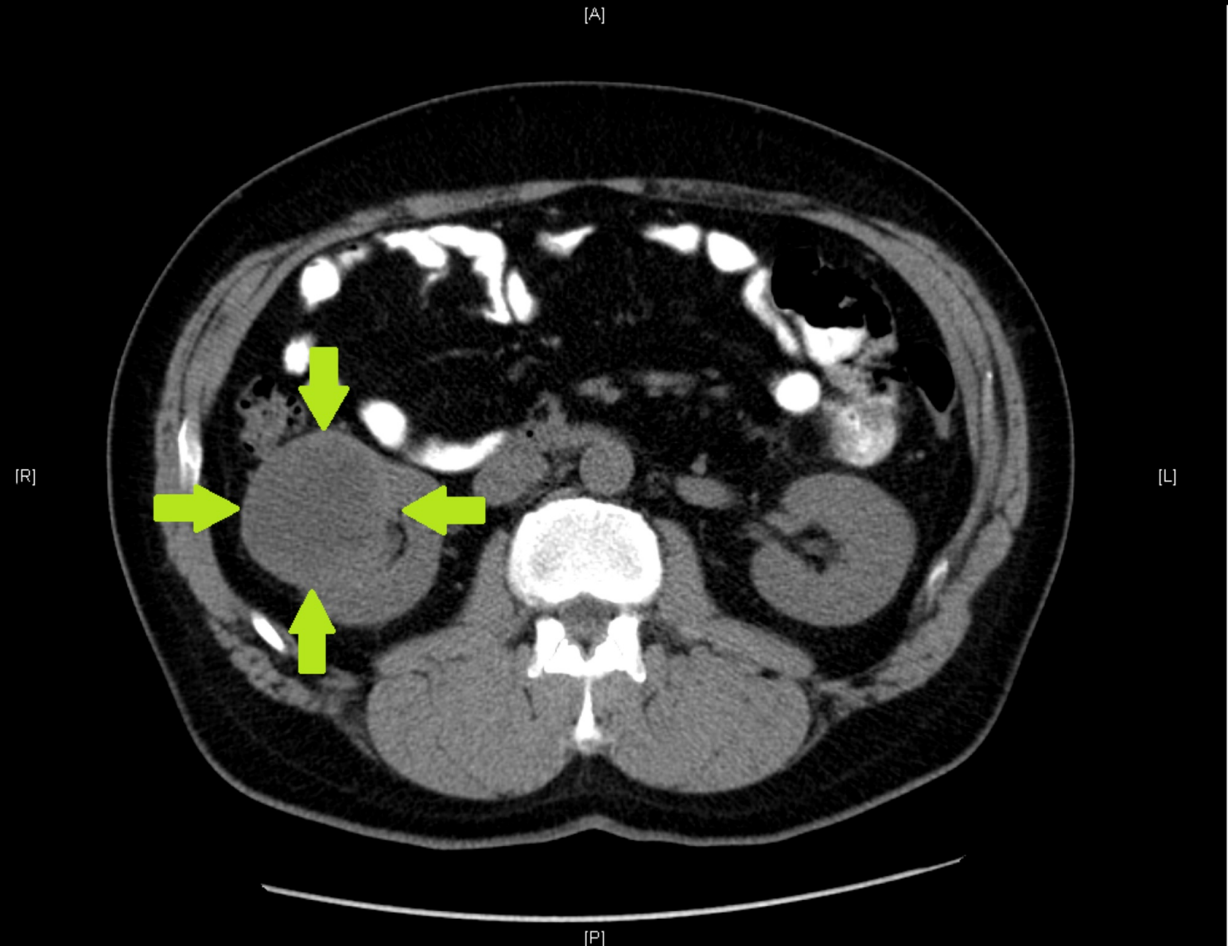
CT abdomen Axial CT image shows large renal cell carcinoma arising from the right kidney.
